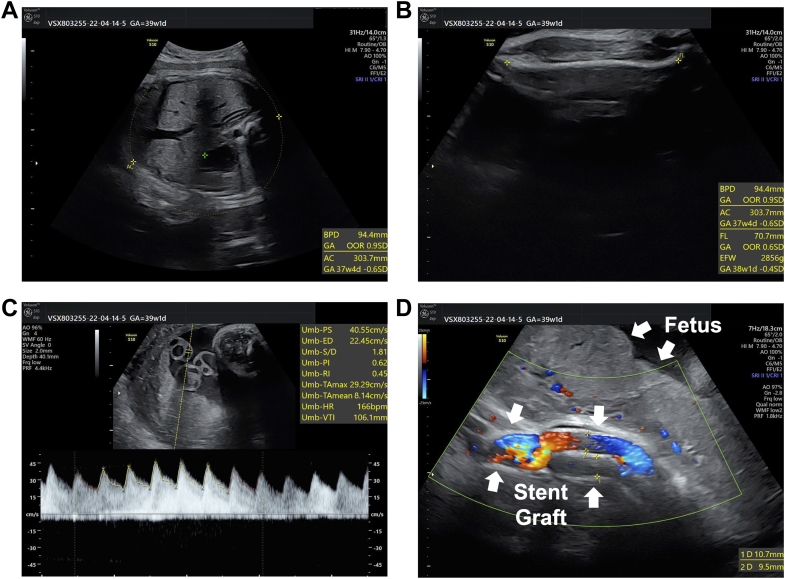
Fetal Ultrasound Findings at 39 Weeks' Gestation (A) Abdominal circumference (303.7 mm). (B) Femur length (70.7 mm). (C) Umbilical artery Doppler tracing obtained on carvedilol 5 mg daily for the patient showed peak systolic velocity 40.6 cm/s, end-diastolic velocity 22.5 cm/s, S/D ratio 1.81, pulsatility index 0.62, resistance index 0.45, and fetal heart rate 166 beats/min. These findings were all within the normal range. (D) Color Doppler image showing the deployed abdominal stent-graft in close proximity to the fetus, with no evidence of compression-induced deformation or graft compromise.
